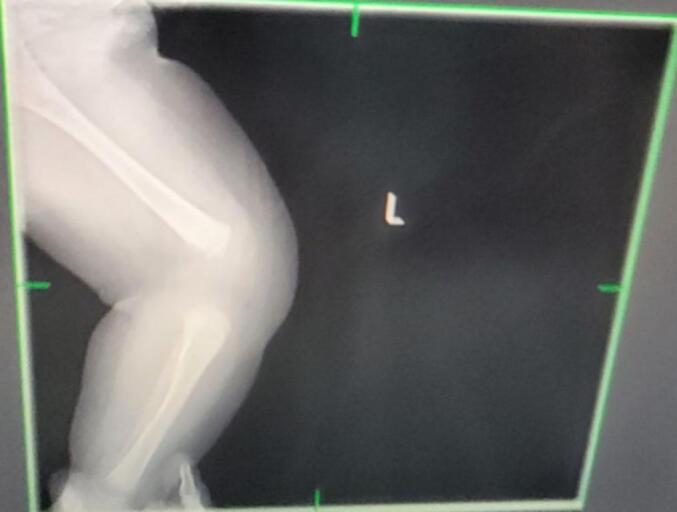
X-ray of left knee showing normal finding.
